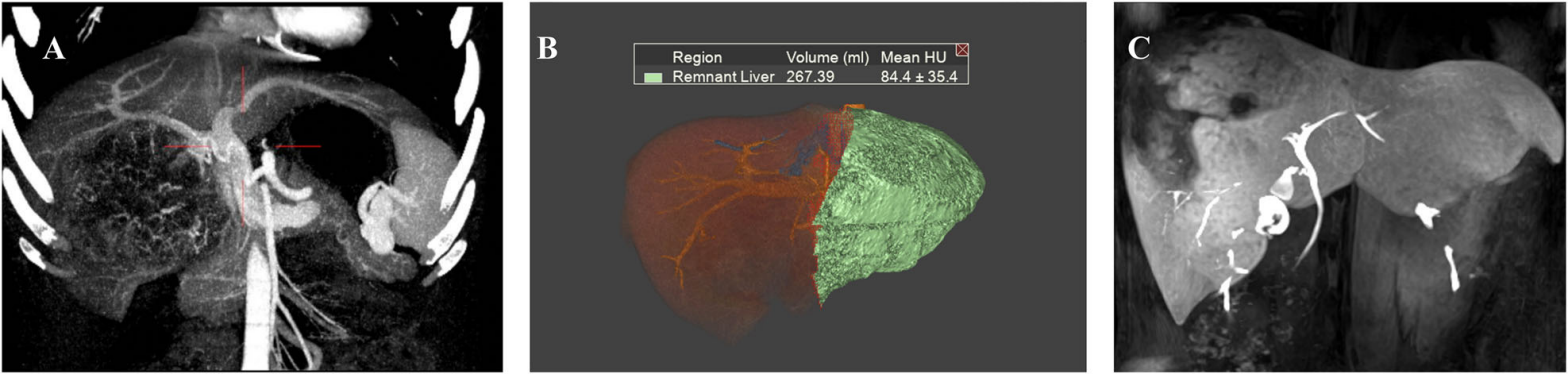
Preoperative assessment with CT and MRI. A The maximum intensity projection of a CT image showed the anatomy of the hepatic artery and portal vein, allowing for the identification of the anatomical variations that may interfere with surgical procedures or cause vascular injury. B A three-dimensional CT image and virtual right hemi-hepatectomy showed the insufficient liver volume of the left lobe. C The maximum intensity projection of an MRI in the hepatobiliary phase showed good liver function and the anatomy of the bile duct: normal contrast enhancement of the hepatic parenchyma, accompanied by normal excretory function of the biliary system
